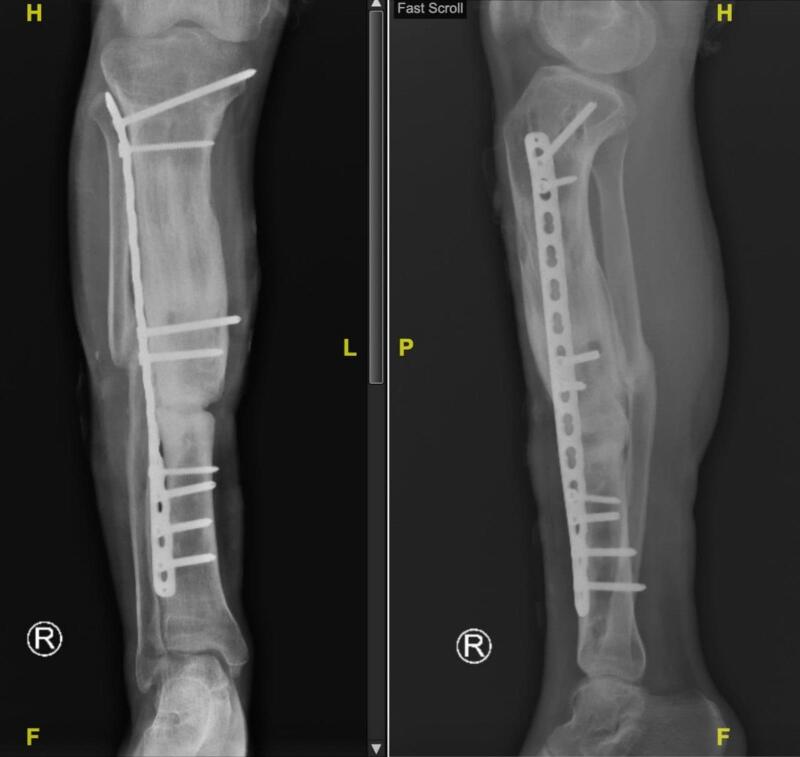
Radiograph images of the leg post ORIF + Bone Graft application in 21 months follow up, anterioposterior (AP) and right lateral (RL) projections of right leg. White arrow: callous formation.
